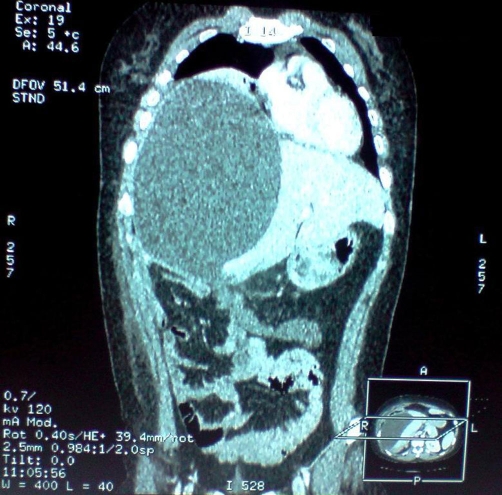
Abdominal CT (coronal) demonstrated unilocular subcapsular fluid-filled collection in the right lobe of the liver.
